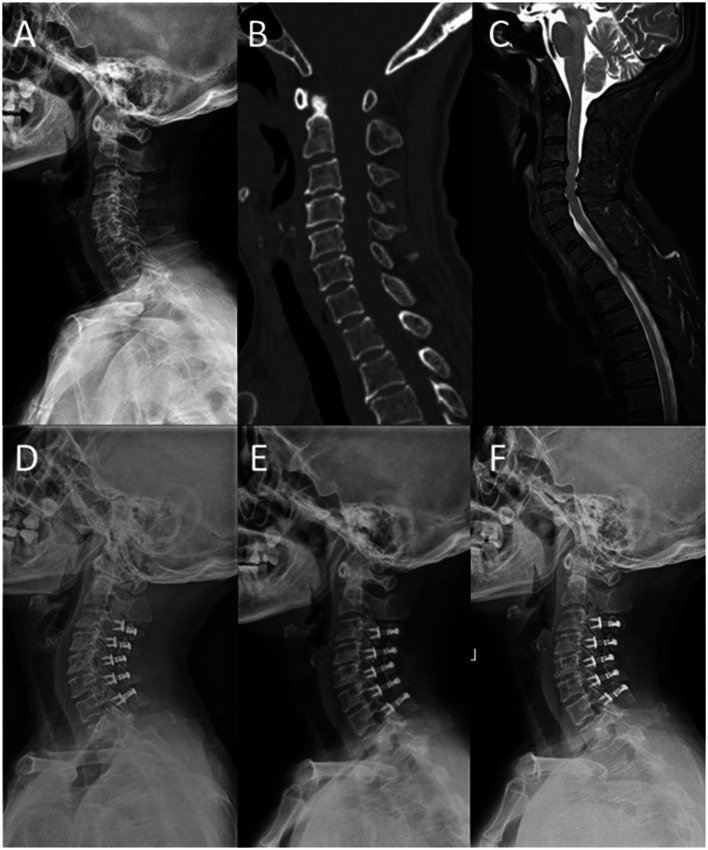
A 65-year-old female was treated with open-door laminoplasty. (A) X-ray lateral view before surgery; (B) sagittal CT scan before surgery; (C) sagittal fat-suppressed sequence in MRI before surgery; (D) postoperative X-ray lateral view; (E) X-ray lateral view at 1 month after surgery; and (F) X-ray lateral view at FFU.
